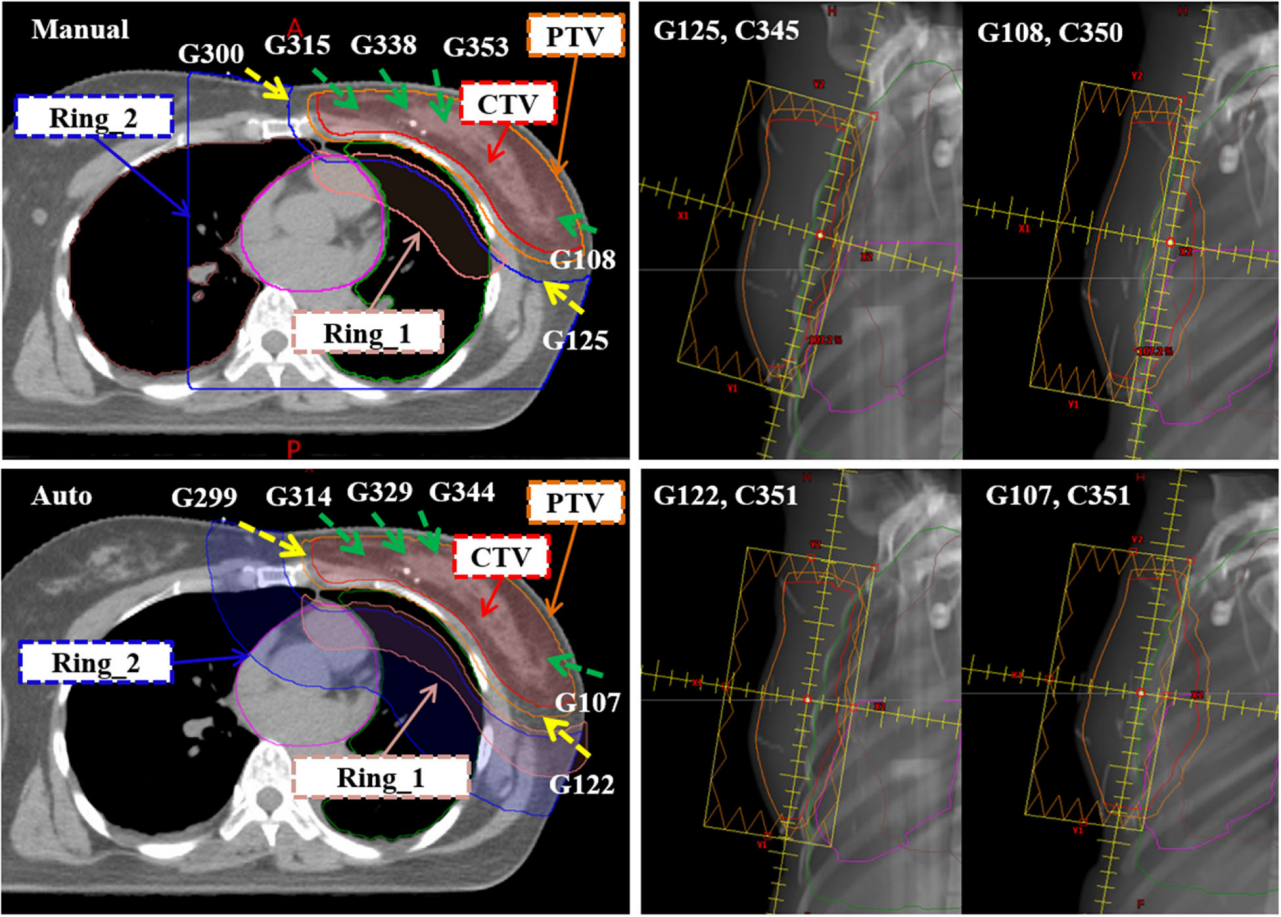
A representative CT axial view demonstrating beam arrangement and planning auxiliary structure (left panel) and beam’s-eye-view (right panel). Upper row: manual plan. Lower row: autoplan
